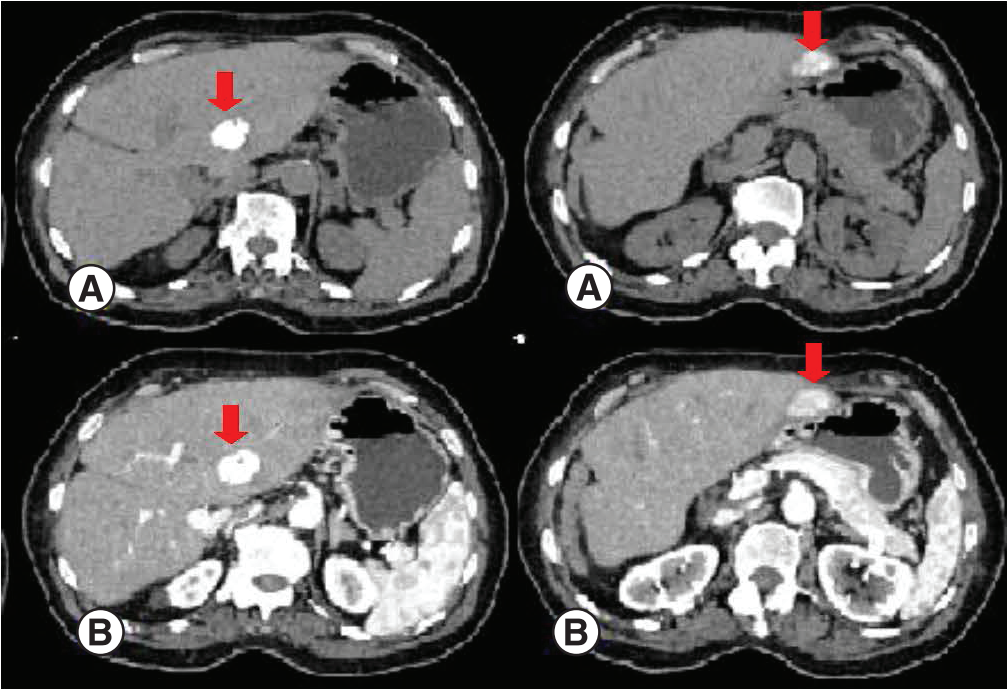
Follow-up abdominal CT taken one month after the second session of transarterial chemoembolization. The CT showed a dense and homogeneous lipiodol deposition in the tumor on precontrast images (red arrow in [A]), without persistent arterial phase hyperenhasement (red arrow in [B]).
